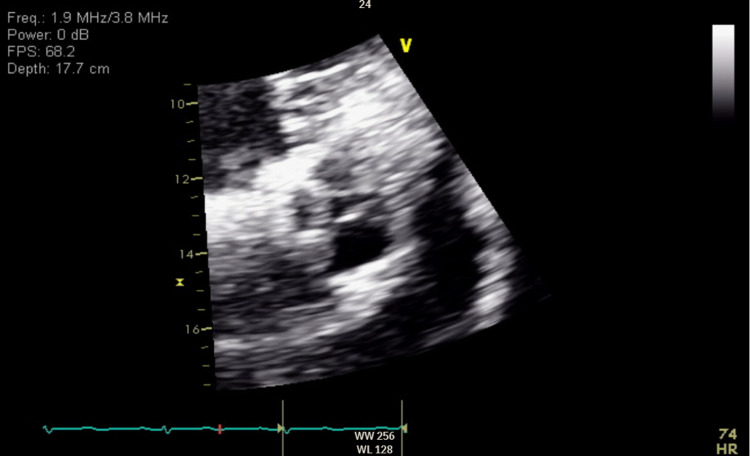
PSAX view zoomed on the aortic valve. PSAX: parasternal short axis.
